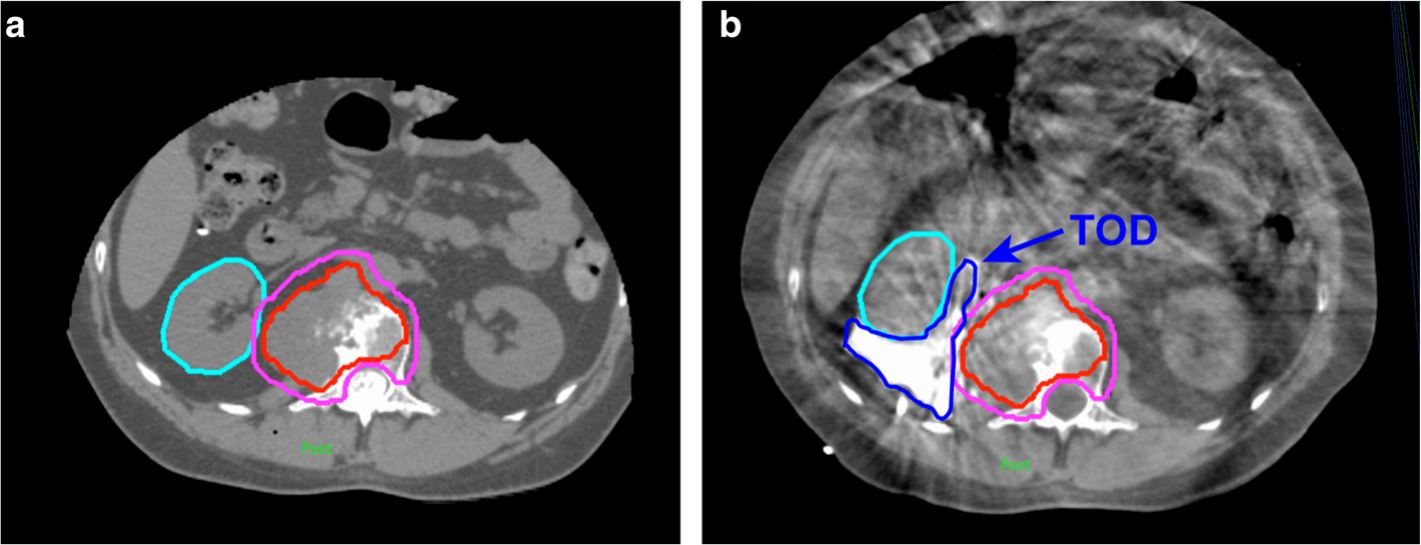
Axial imaging of paraspinal non-seminomatous germ cell tumor treated to 2850 cGy. (a) Baseline computed tomography (CT) scan revealing paraspinal target lesion adjacent to the kidney at L1. (b) Cone-beam CT confirming TOD kidney.
